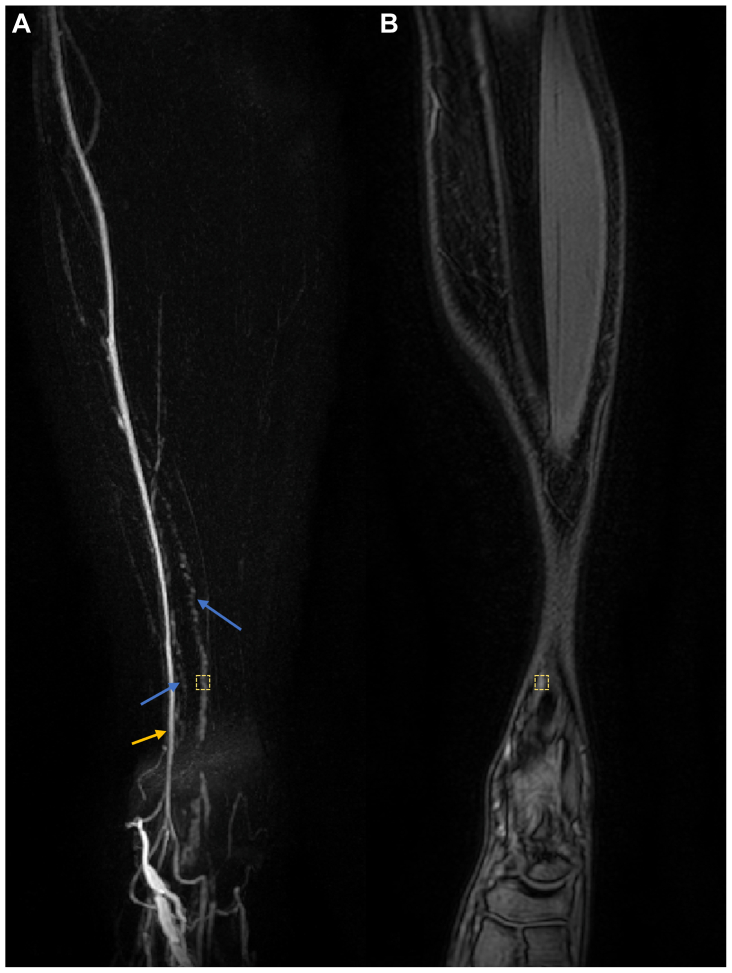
Lymphatic vessel anatomy and function can be visualized. Left leg of a 25-year-old woman showing lymphatic (*blue arrows*) and venous (*orange arrow*) vessels with T1-weighted spoilt gradient echo imaging approximately 20 minutes after contrast administration. A, A maximum intensity projection (MIP) is shown after subtraction of the first post-contrast image. B, A single coronal image slice of the same timepoint with the approximate location at which lymphatic signal was measured (shown with an *orange hashed box*; enlarged for better visibility).
